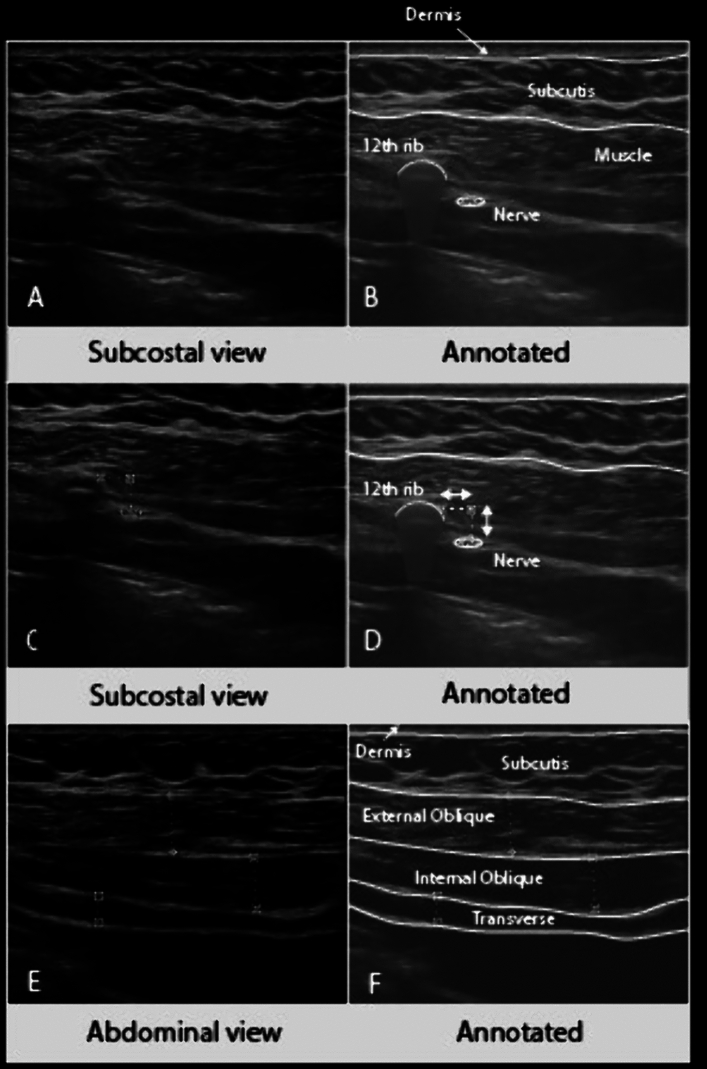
Examples of preoperative high-resolution ultrasound imaging. A, B Cross-sectional view of the subcostal nerve at the tip of the 12th rib. C, D Distance of the subcostal nerve to the tip of the 12th rib. (E, F) Diameters of the abdominal wall muscles at the posterior axillary line
